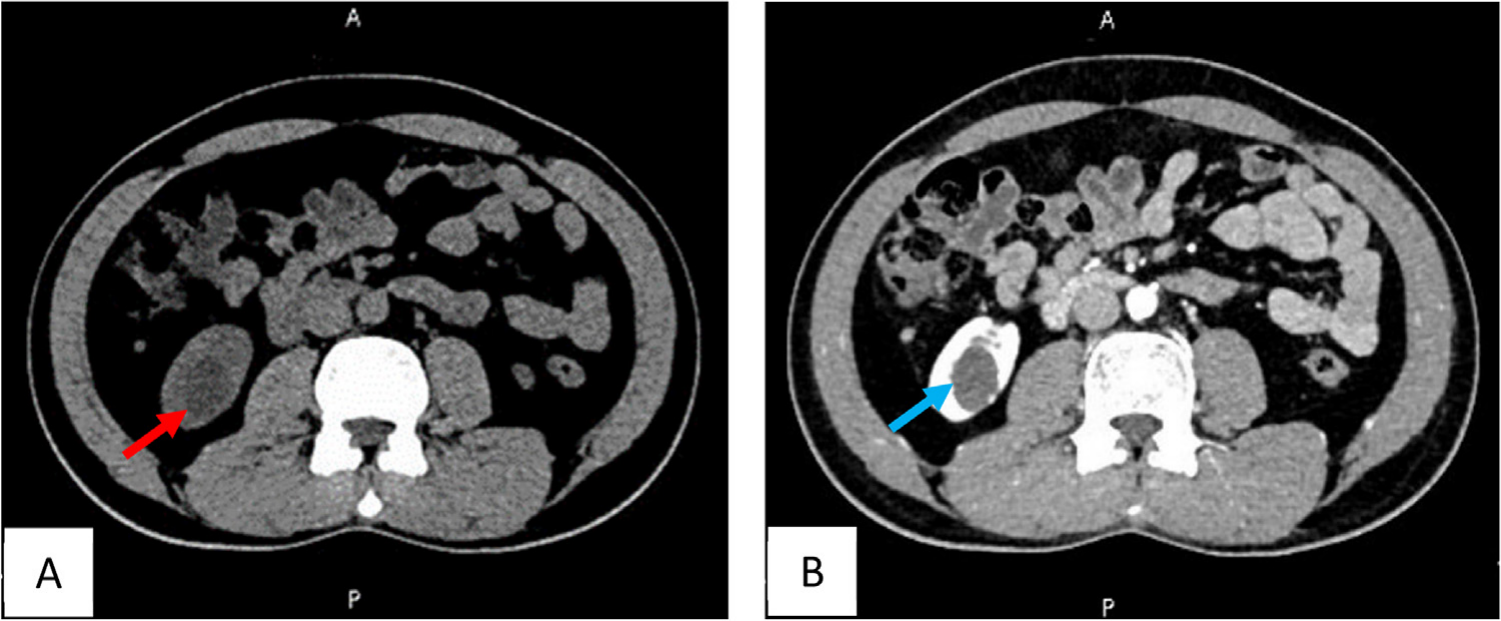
CT scan of the right renal lesion. (A) non contrast CT showing homogenous and hypodense lesion (red arrow), (B) no enhancement seen after IV contrast (blue arrow).
